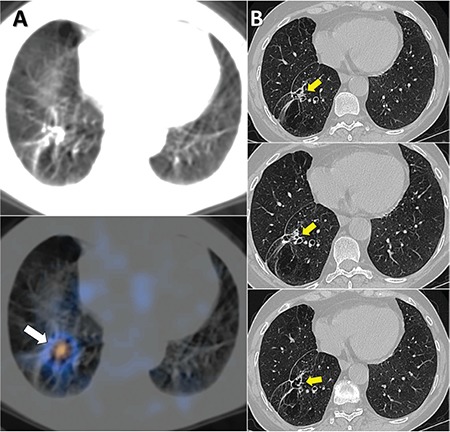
SPECT/CT images showed focal I-131 uptake at the wedge-resection site (A). A diagnostic CT was performed due to the possibility of residual lesion. Sequential slices demonstrated only focal traction bronchiectasis due to wedge-resection (B). A few case reports showed incidental detection of I-131 uptake in bronchiectasis (1,2,3). However, this case was different because we observed focal uptake at the metastasectomy site, which could be thought to be due to a residual lesion. Since the CT component of our SPECT/CT was not enough to clarify this issue, a diagnostic CT was obtained.
